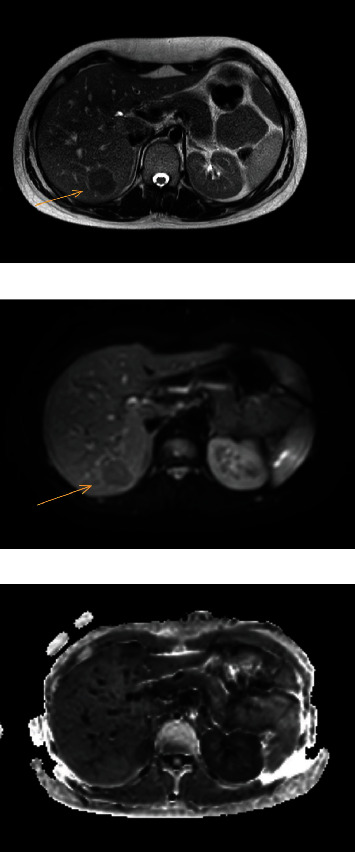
The lesions were isointense in T1-weighted images and hyperintense in T2-weighted images and did not demonstrate any diffusion restriction.
